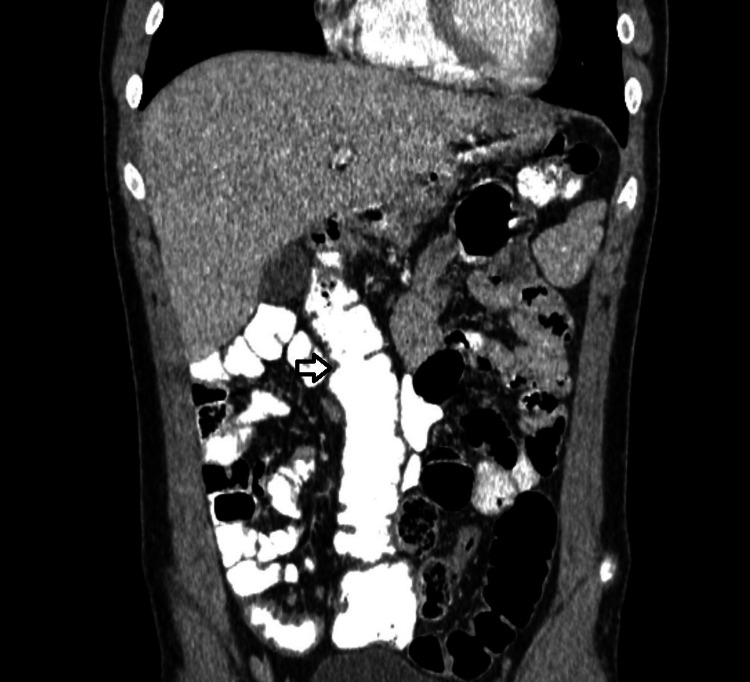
Enhanced CT with oral contrast, coronary view showing the ascending colon (white arrow) lying in the midline and the small intestine, located at the right side of the abdomen
